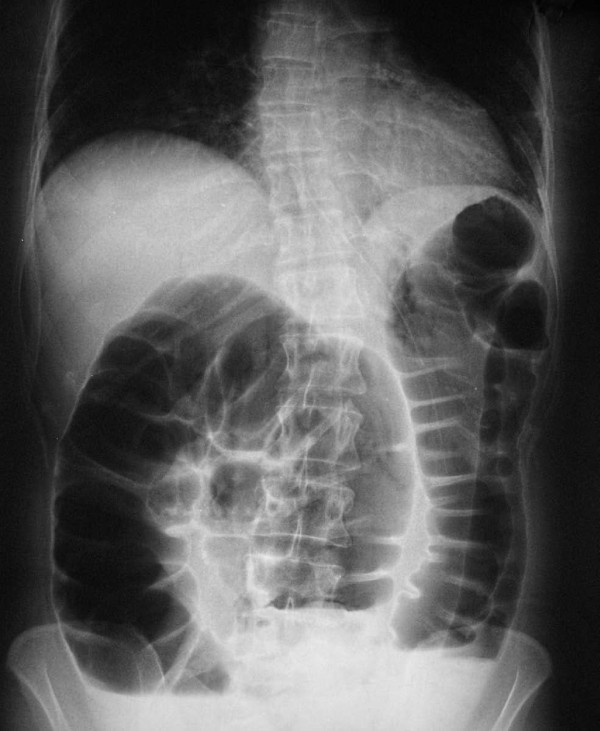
Abdominal X-ray shows dilatation of proximal bowel segments.
